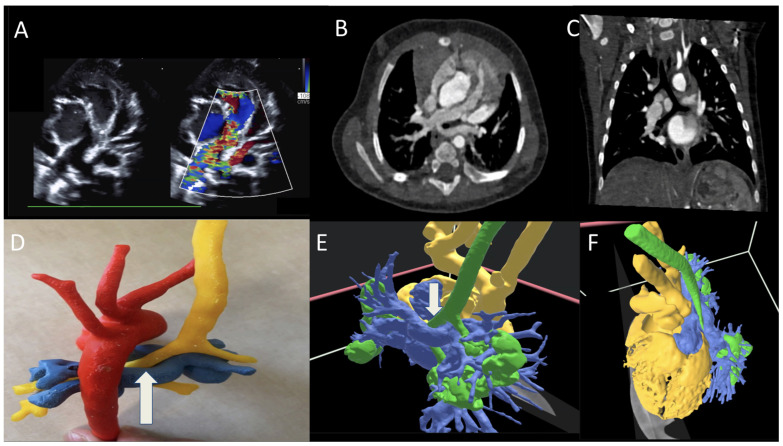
(A) shows an 2D and color echocardiogram of the LPA sling; (B,C) are CT; (D) is a 3D-printed model of the LPA sling with trachea in yellow which is narrowed. Tracheal bronchus. Accessory bronchus originates directly from the trachea; (E,F) are VR images with an arrow pointing towards the LPA sling and trachea in green.
